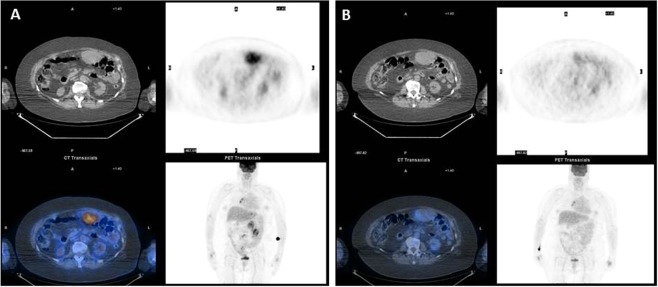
(A) The basal functional imaging by FDG-PET before starting imatinib revealed a pathological FDG up-take corresponding with several abdominal lesions. (B) Functional imaging by FDG-PET after one month of treatment revealing a complete metabolic response of all lesions.
